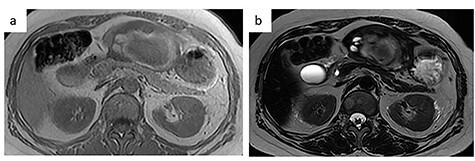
MRI. (a) T1-weighted image: low-intensity area suggestive of intratumor hemorrhage. (b) T2-weighted image: the tumor had heterogenous intensity.
